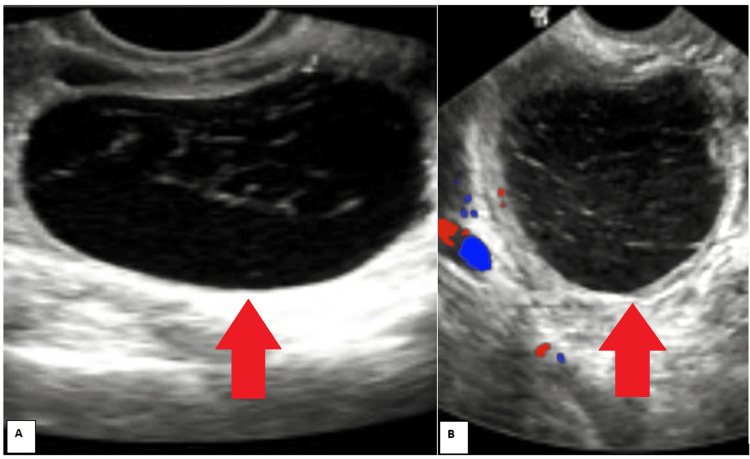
Preoperative ultrasound: (A) cystic lesion in the pelvis; (B) cystic lesion showing absence of vascularity (torsion).
